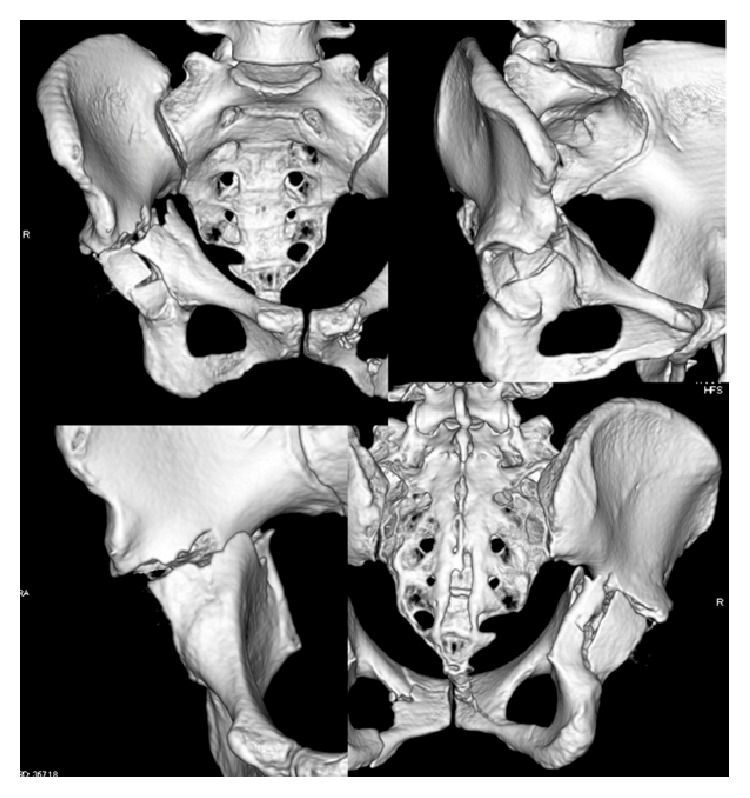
Three-dimensional computed tomography images showing a transverse fracture and posterior wall fracture of the right acetabulum.
